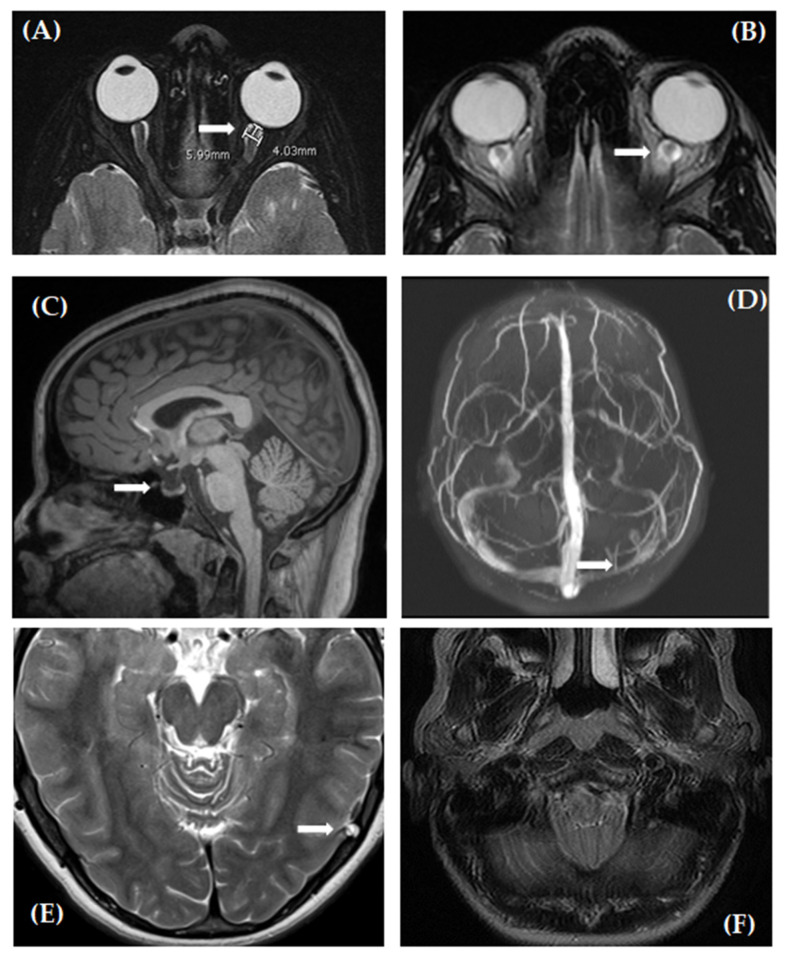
Neuroimaging examples from IIH patients. Axial fat-suppressed T2-weighted image of the orbits (A) demonstrates intraocular protrusion of the optic nerve head bilaterally and distension of the perioptic subarachnoid space, as measured 4 mm posterior to the globe perpendicular to the long axis of the optic nerve, on the left (arrow). In this example, the diameter measured 5.99 mm as shown. Axial T2-weighted image of the orbits (B) demonstrates flattening of the posterior contour of the globes bilaterally. The perioptic subarachnoid space is distended (arrow). Sagittal T1-weighted MPRAGE image of the brain (C) demonstrates a partially empty sella turcica (arrow). Maximum intensity projection (MIP) image from a coronal 2D TOF MRV (D) demonstrates segmental stenosis or hypoplasia of the left transverse sinus (arrow). Axial T2-weighted image of the brain (E) demonstrates a prominent arachnoid granulation (arrow). Axial T2-weighted image of the brain (F) at the level of the foramen magnum demonstrates cerebellar tonsillar ectopia with crowding.
